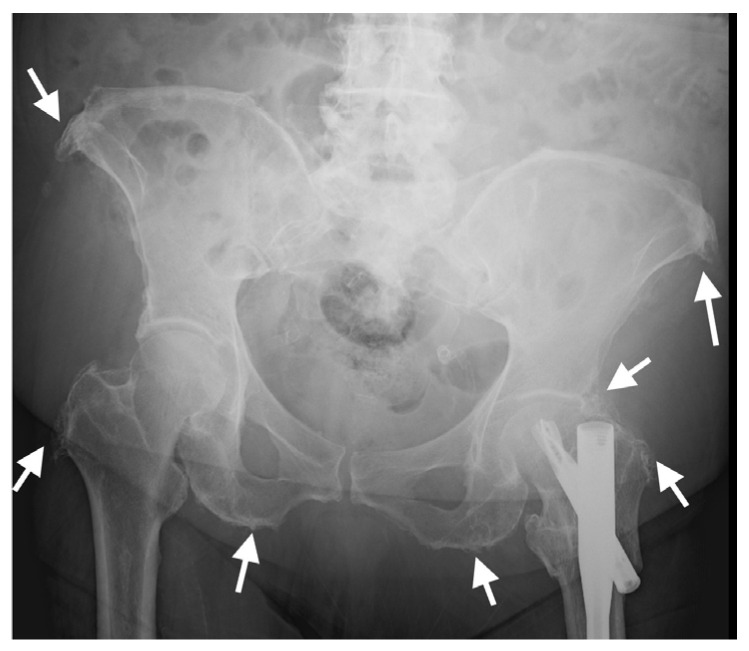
AP radiograph of the pelvis of an 82-year-old female with thoracic DISH (not shown). Characteristic whiskering of the anterior superior iliac spine and ischial tuberosities (arrows) is present on both sides, resulting from enthesopathy in these regions.
